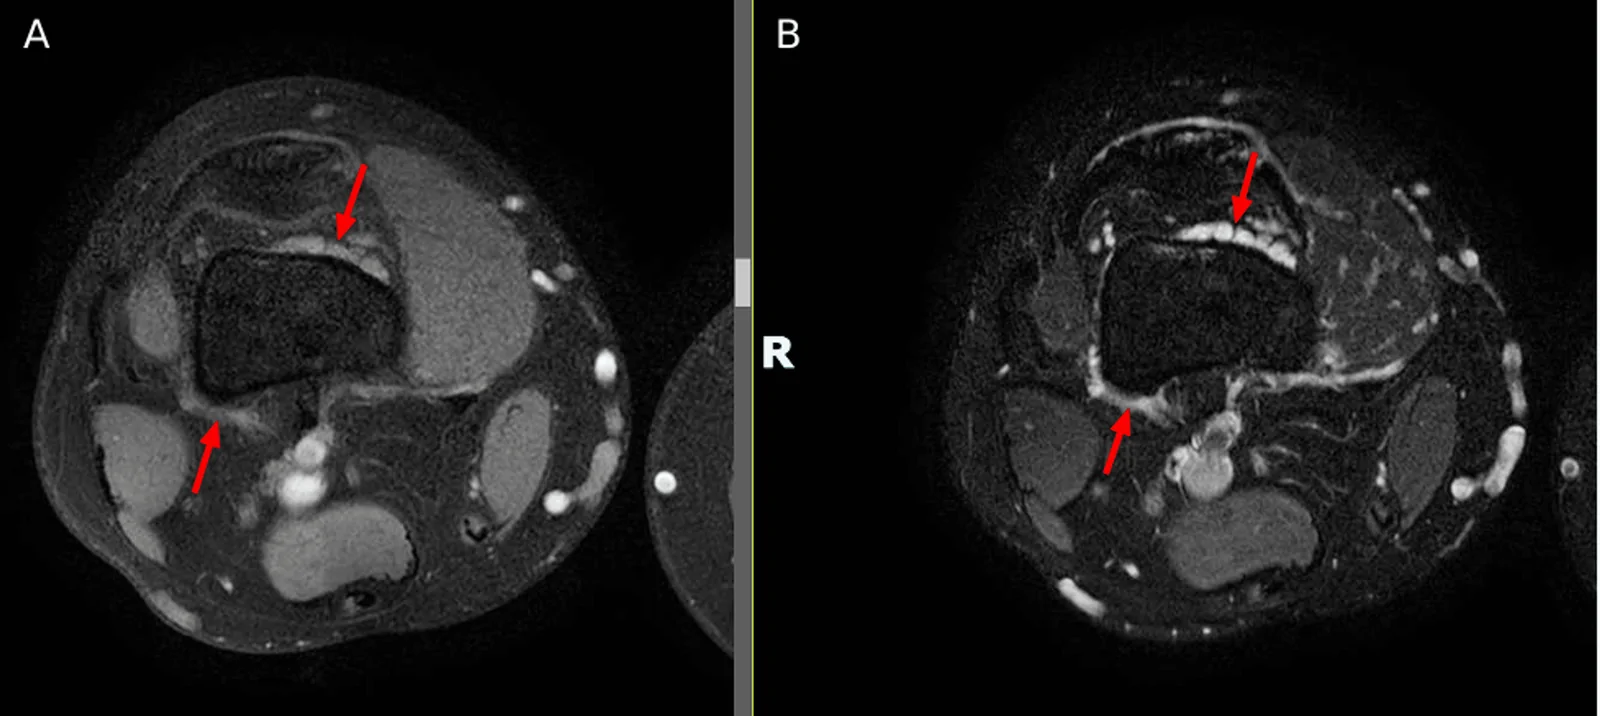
MRI of the right knee demonstrating features of a venous malformation. (A) Intermediate signal intensity on T1-weighted imaging. (B) Hyperintense signal on T2-weighted imaging, consistent with the characteristics of venous malformations.
